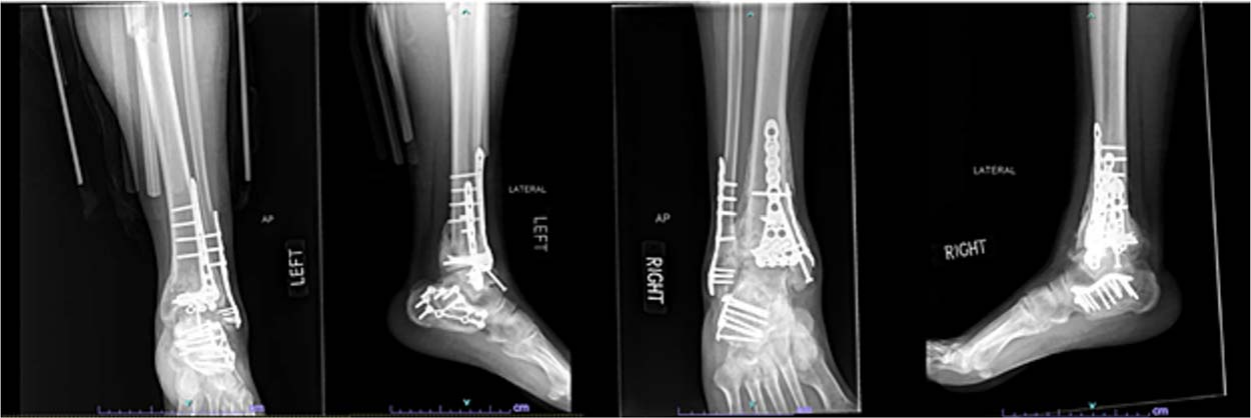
Two-year follow-up AP and lateral radiographs of both ankles in patient 2 showing maintained alignment and hardware position.
